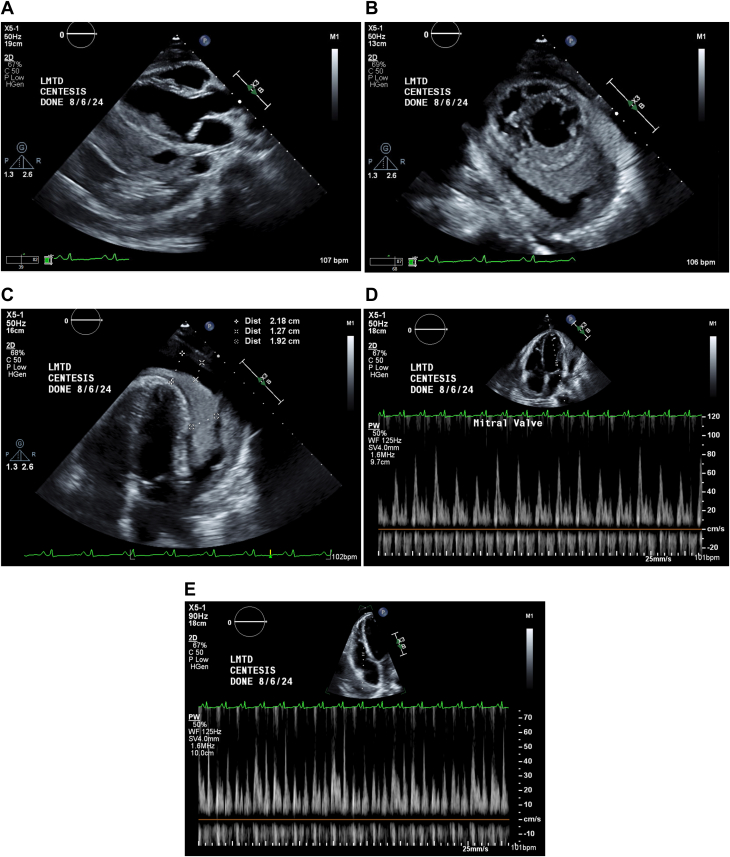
Postprocedural Echocardiography Repeat transthoracic echocardiogram imaging day 1 after pericardiocentesis and drain placement (A-C) with multiple windows displaying persistent complex fluid collection concerning for hemodynamic comprise (D, E) despite adequate placement and function.
